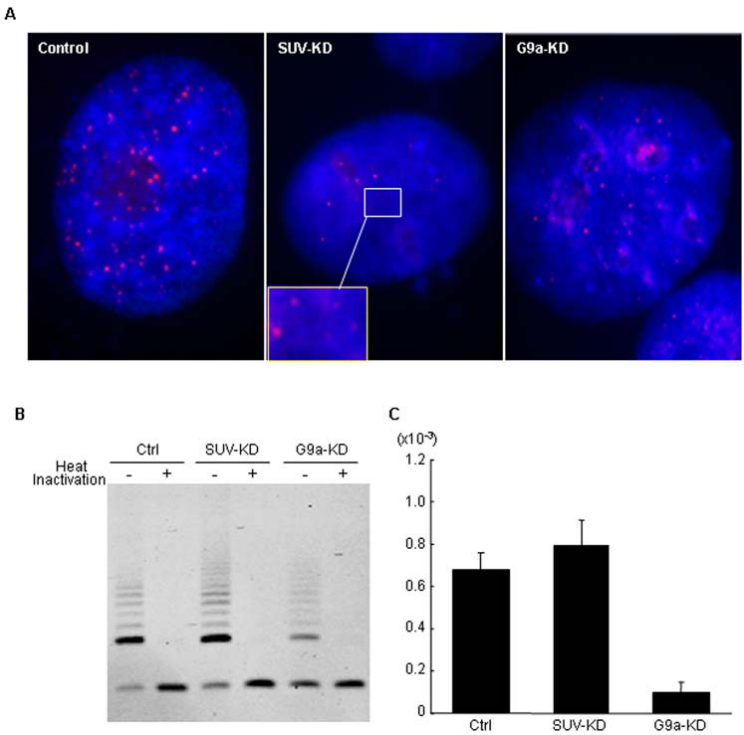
Disruptions of telomere function in the G9a-KD and the SUV-KD cells. A, Interphase nuclei were hybridized with Cy3-conjugated peptide nucleic acid (PNA) telomeric probe and counterstained with DAPI. Marked reduction in the telomere signal is observed in interphase nuclei from KD cells as compared to the nuclei from the control line. Shown in the insets are the enlarged images of the indicated areas. B, Telomerase activity is assessed by TRAP assay. Samples with telomerase activity produce a 6-base incremental ladder of TRAP products on the gels. Internal control bands appear at the bottom of the gel. A reduced signal intensity of telomerase activity is observed in the G9a-KD cell lines. The signal is not observed when the extracts are pretreated with heat to abolish the protein components of telomerase, suggesting the telomerase specificity of the signal measured by this assay. C, Expression level of hTERT was measured by real-time PCR. hTERT expression is decreased in the G9a-KD cells compared to those in the control PC3 and the SUV-KD cells. Error bars indicate standard deviation of the 3 independent assays.
